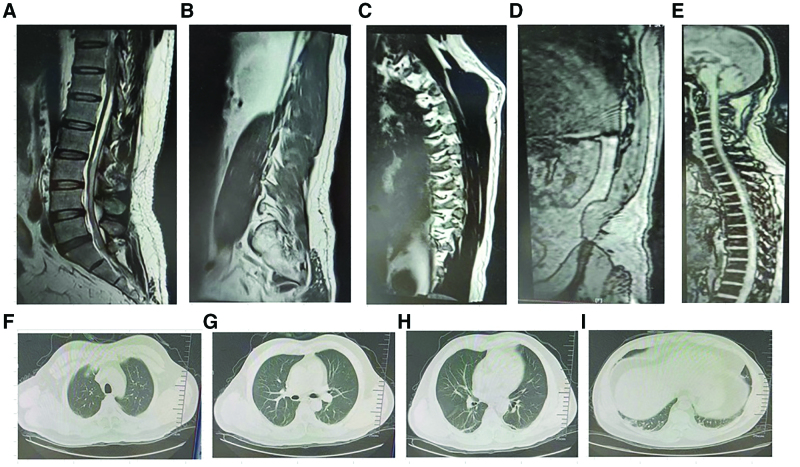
Changes in the chest computed tomography (CT) and magnetic resonance imaging (MRI) of the thoracic and lumbar spine at the time of patient admission. (A–E) Changes in the thoracic and lumbar spine MRI at the time of patient admission. (F–I) Changes in chest CT of the patient upon admission.
